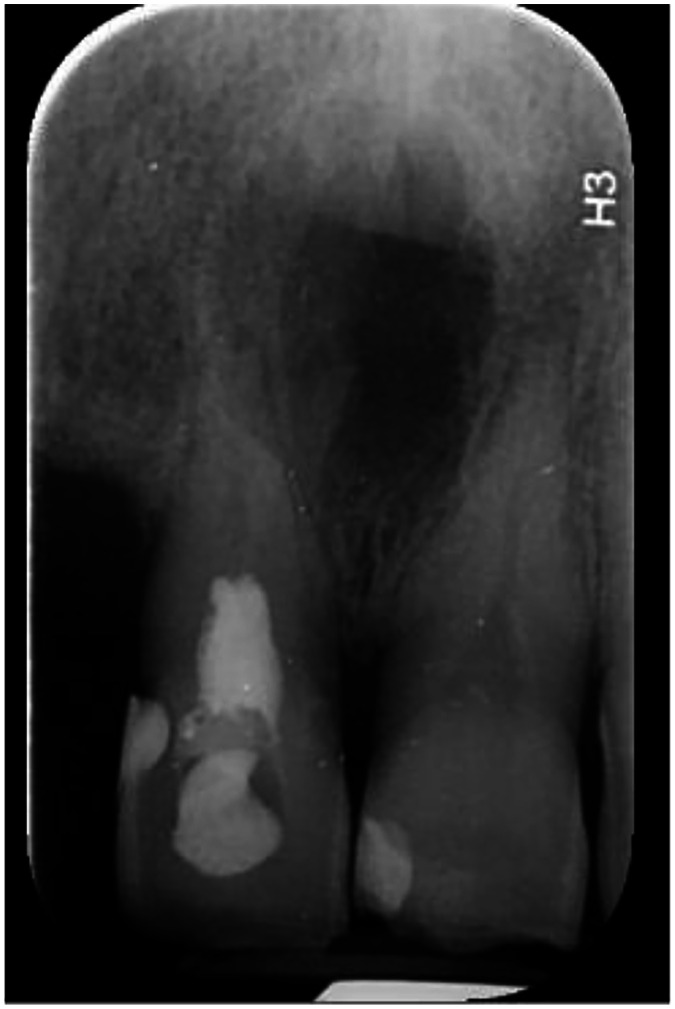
Three-month follow-up Periapical radiography.
